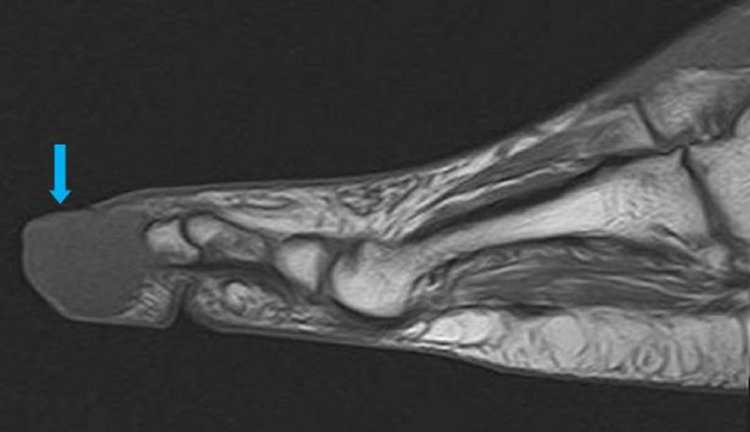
Sagittal view on the MRI T1 sequence visualizing the mass on the distal phalange of the fourth toe (blue arrow)
